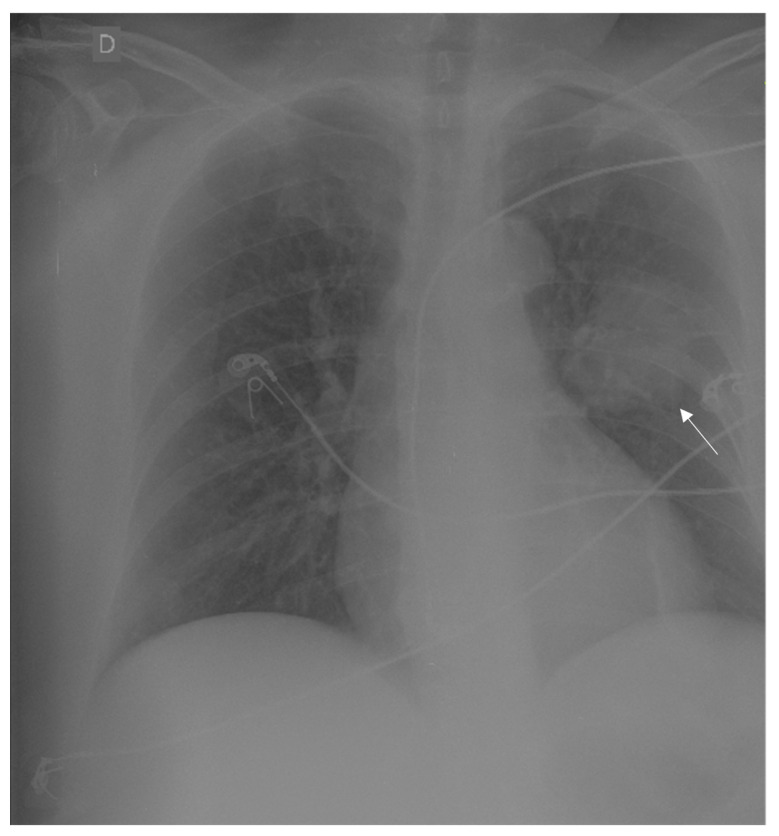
Chest X-ray showing a round mass in the upper lobe of the left lung (indicated with an arrow) suggestive of lung cancer (D in the image means right side).
